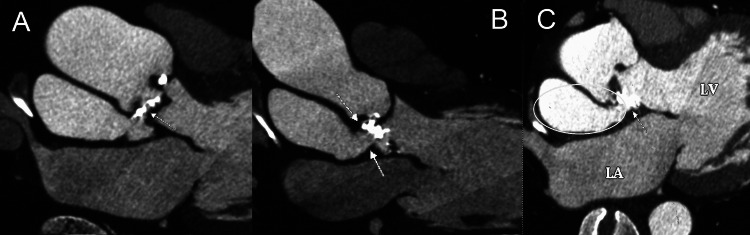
CT coronary angiography A: Blood-filled cavity posterior to the aortic root communicating with the aortic root via a neck measuring 4.3 mm and a heavily calcified aortic valve (arrow); B: Another blood-filled cavity noted posterior to the aortic root communicating with the LVOT through a neck measuring 8 mm; C: Blood-filled cavity of size 5.3 x 5.4 x 2.3 mm posterior to the aortic root and ascending aorta. Posteriorly, the cavity closely abuts the left atrium without any significant compression, with no communication noted with the left atrium. LVOT: Left ventricular outflow tract
